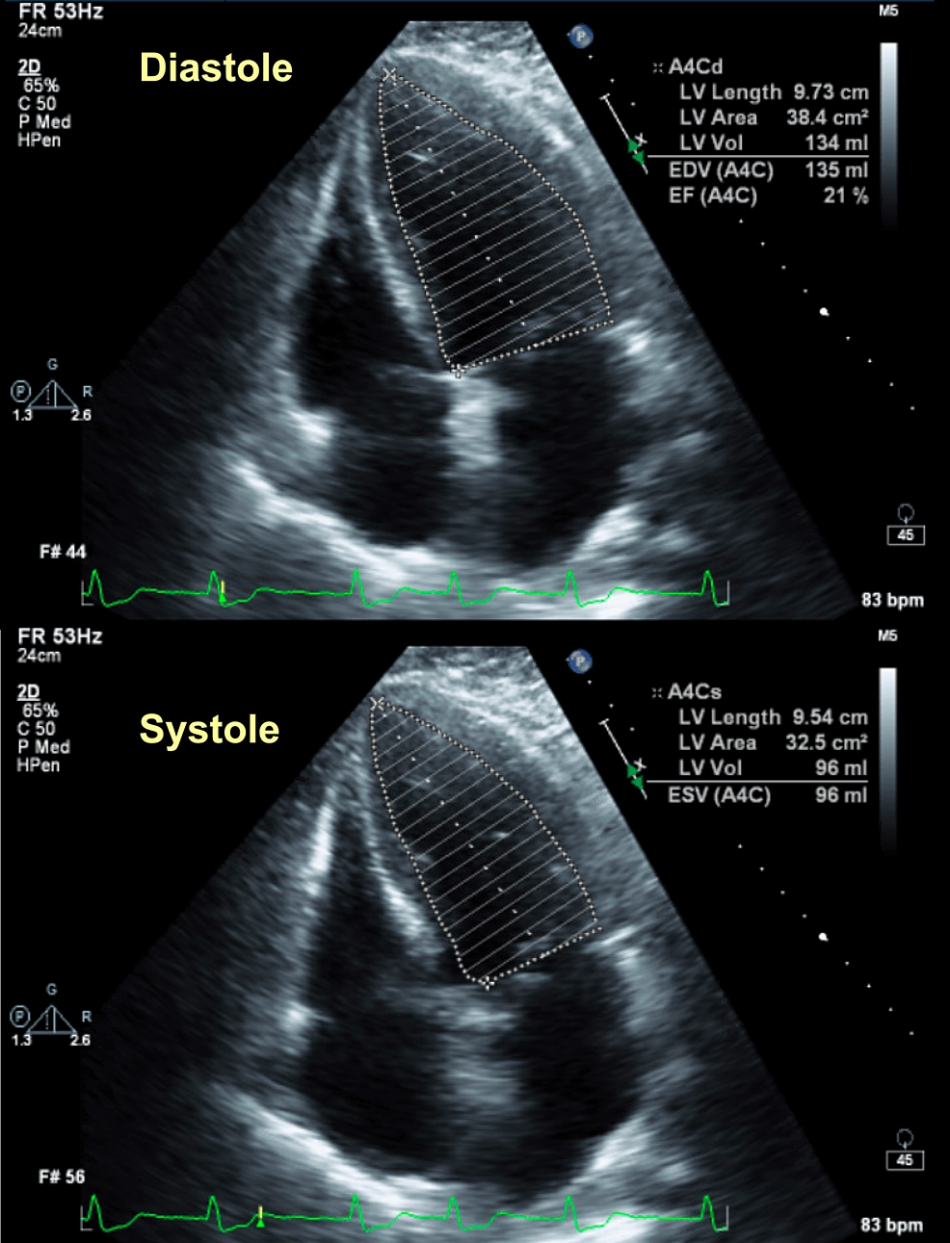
A TTE of the patient during his first admission showing evidence of acute HF with an estimated EF of 15-20%. The EF was measured by a cardiologist and the numbers shown here were inserted by the technician before being reviewed by the cardiologist TTE, transthoracic echocardiogram; HF, heart failure; EF, ejection fraction
